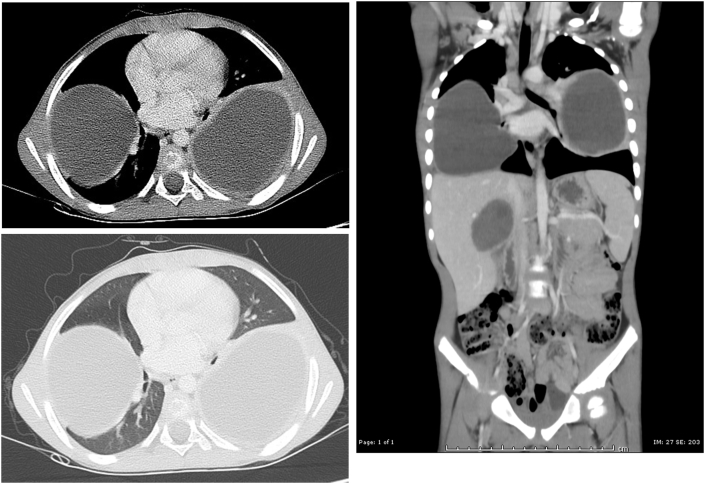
CT of the chest shows intact round, homogeneous, water-density with enhancing rim. (A) Axial mediastinal window, (B) Axial pulmonary window, (C) Coronal mediastinal window showing bilateral intact pulmonary and liver hydatid cysts.
